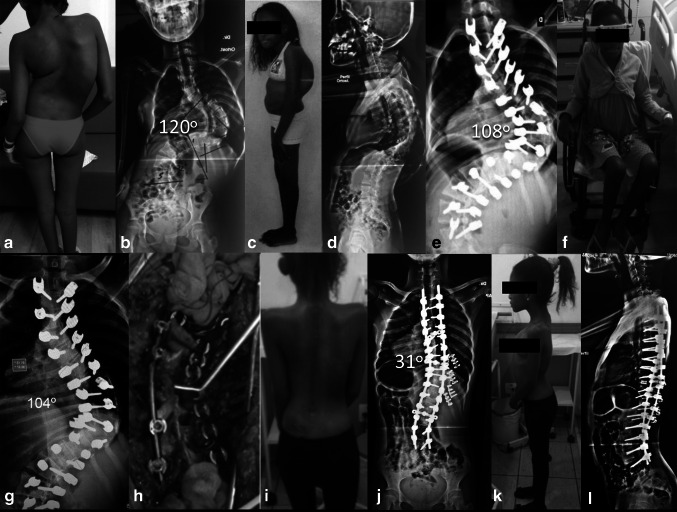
11-year-old female with severe rigid juvenile idiopathic scoliosis. Patient underwent preoperative halo-gravitational traction followed by staged procedures. a–d Preoperative clinical and radiographic images. e, f First, posterior release and instrumentation and postoperative halo-gravitational traction. g Radiograph showing poor correction with traction. h–l Second-stage posterior vertebral column resection and thoracoplasty reconstruction with internal osteosynthesis (TRIO)
